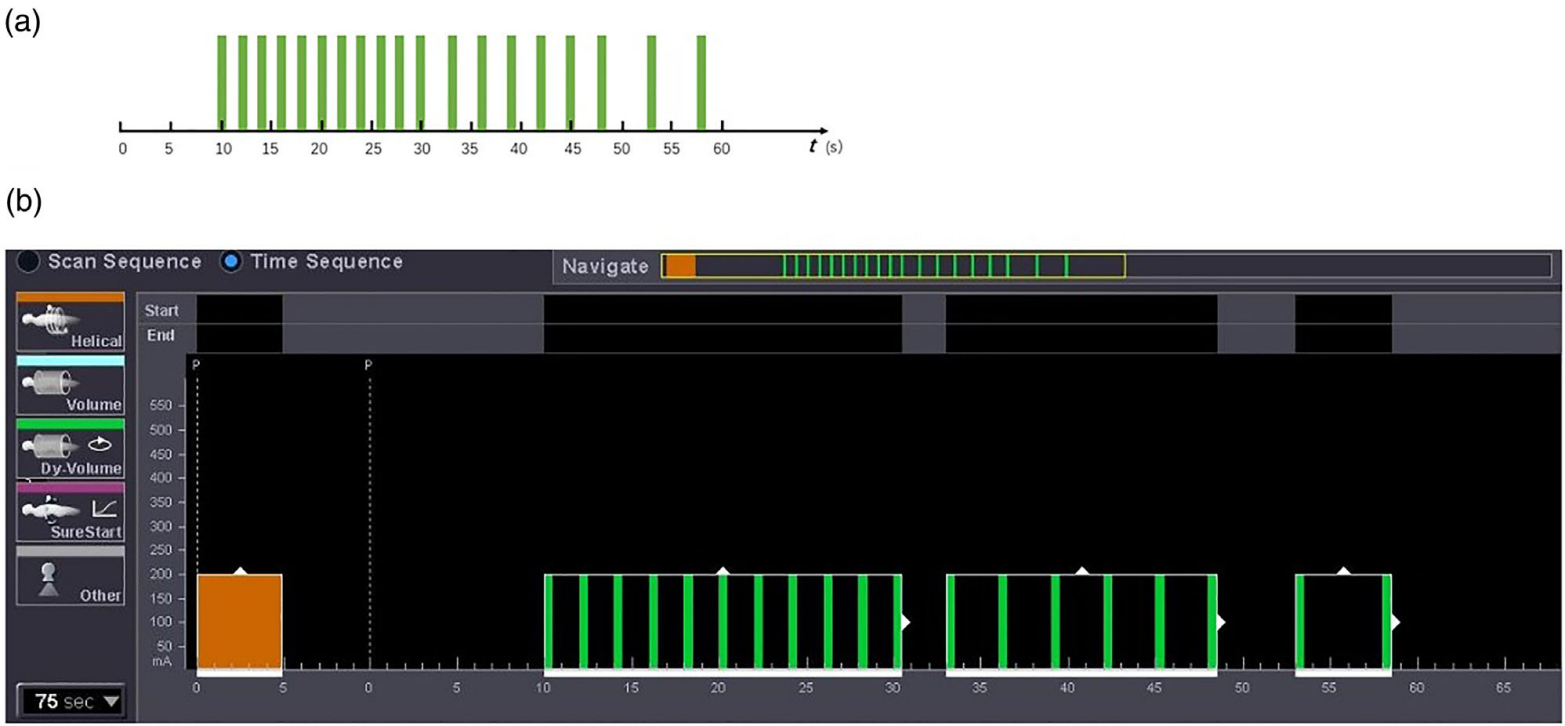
(a and b) The detailed scanning protocol of pancreatic computed tomography perfusion (CTP)
